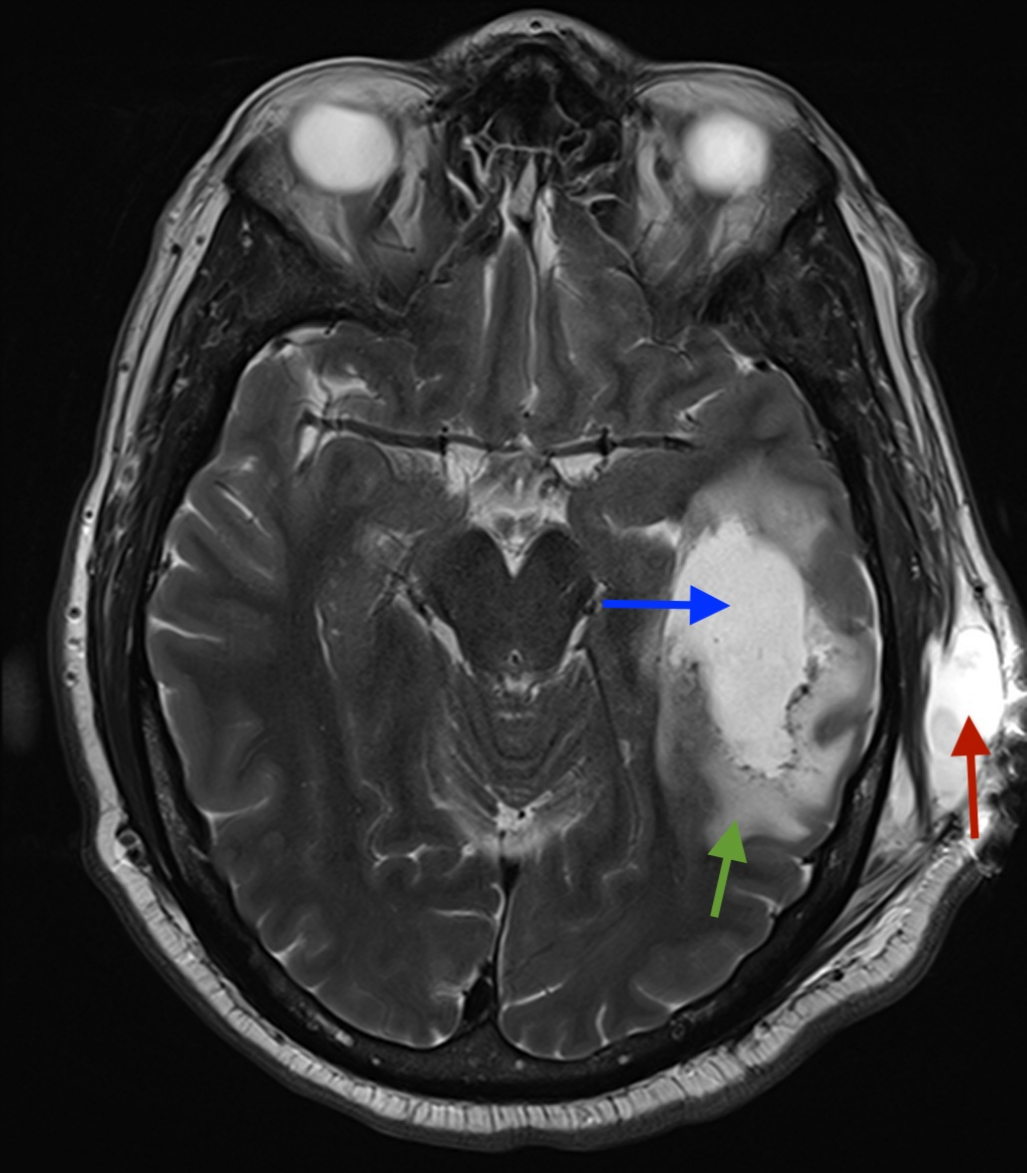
Axial unenhanced T2 MRI of the brain: lucency in temporal lobe (blue arrow) and associated surrounding oedema (green arrow), pseudomeningocoele (red arrow) in subcutaneous tissues on the left side.
